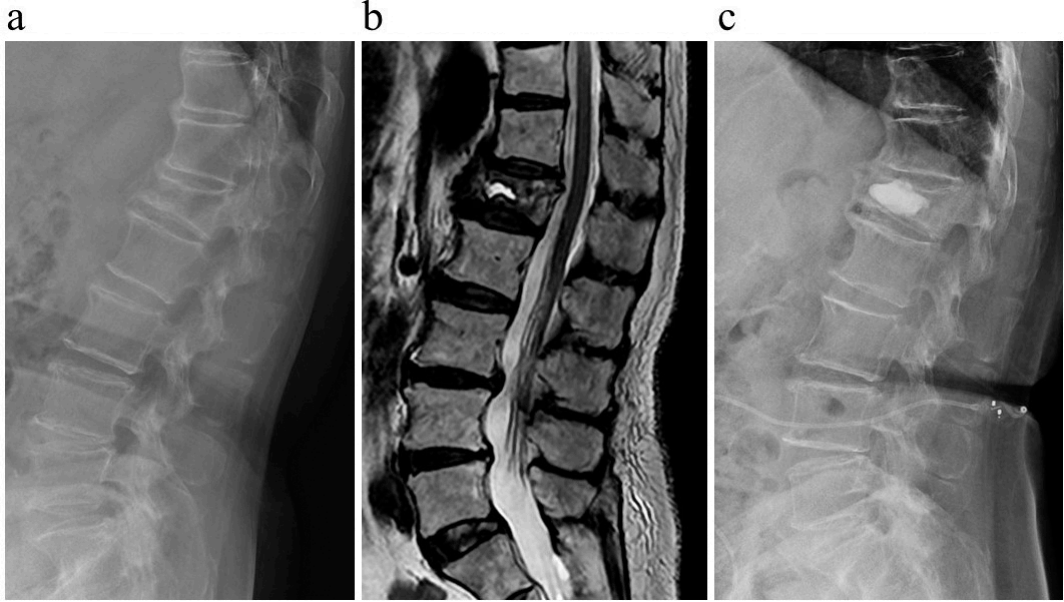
Preoperative plain spinal radiographs demonstrated L1 compression fracture (a). T2-weighted imaging on magnetic resonance imaging revealed a recent L1 vertebral compression fracture without spinal canal compromise (b). Plain spinal radiographs after balloon kyphoplasty reveal effective filling of cystic fracture cavity (c).
